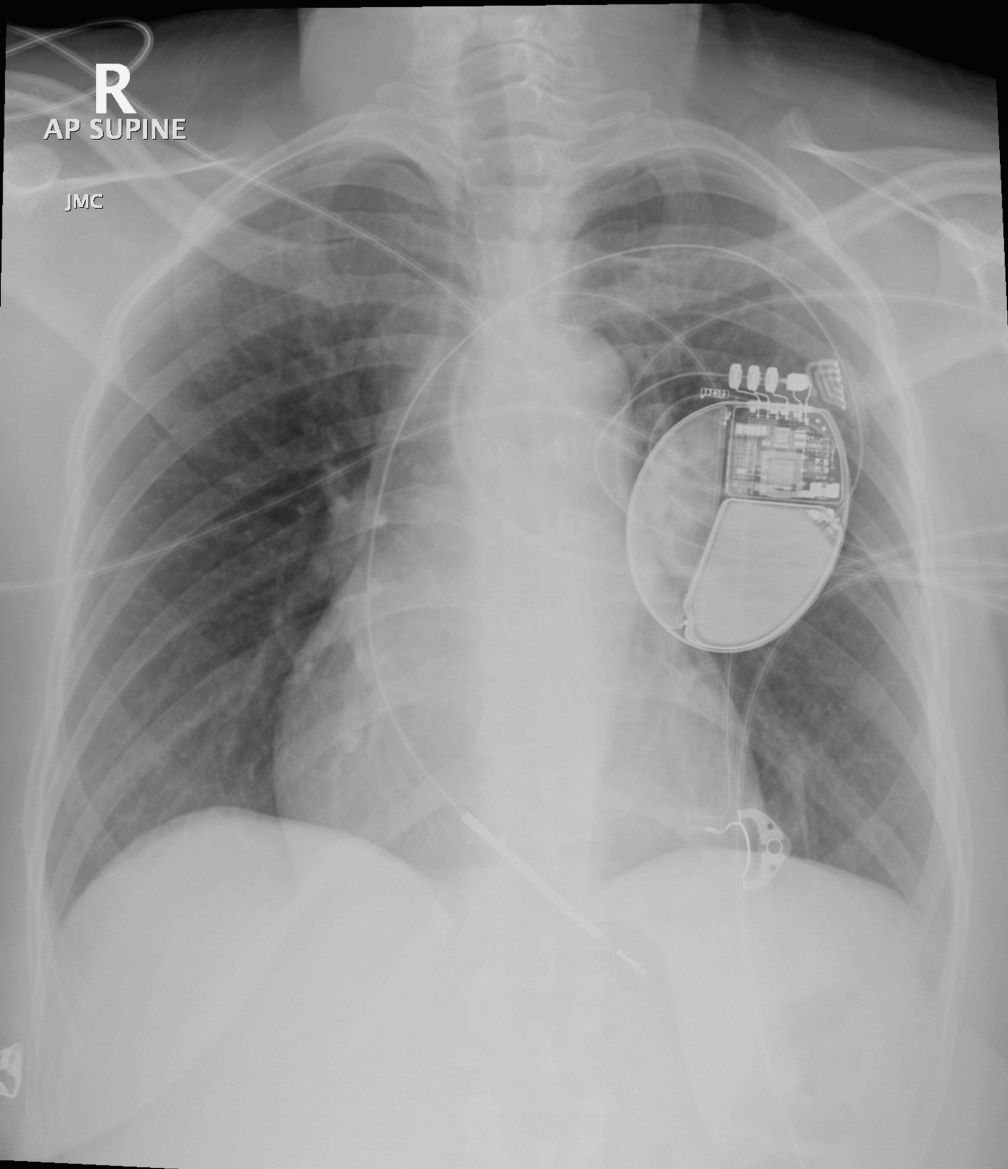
Mobile chest X-ray, anteroposterior (AP) view in supine position AP supine chest X-ray shows a dual-chamber implantable cardioverter-defibrillator (ICD) in the left pectoral area with well-positioned leads. Lungs are clear with no pneumothorax or lead issues. Overall, ICD placement appears satisfactory with no immediate complications.
